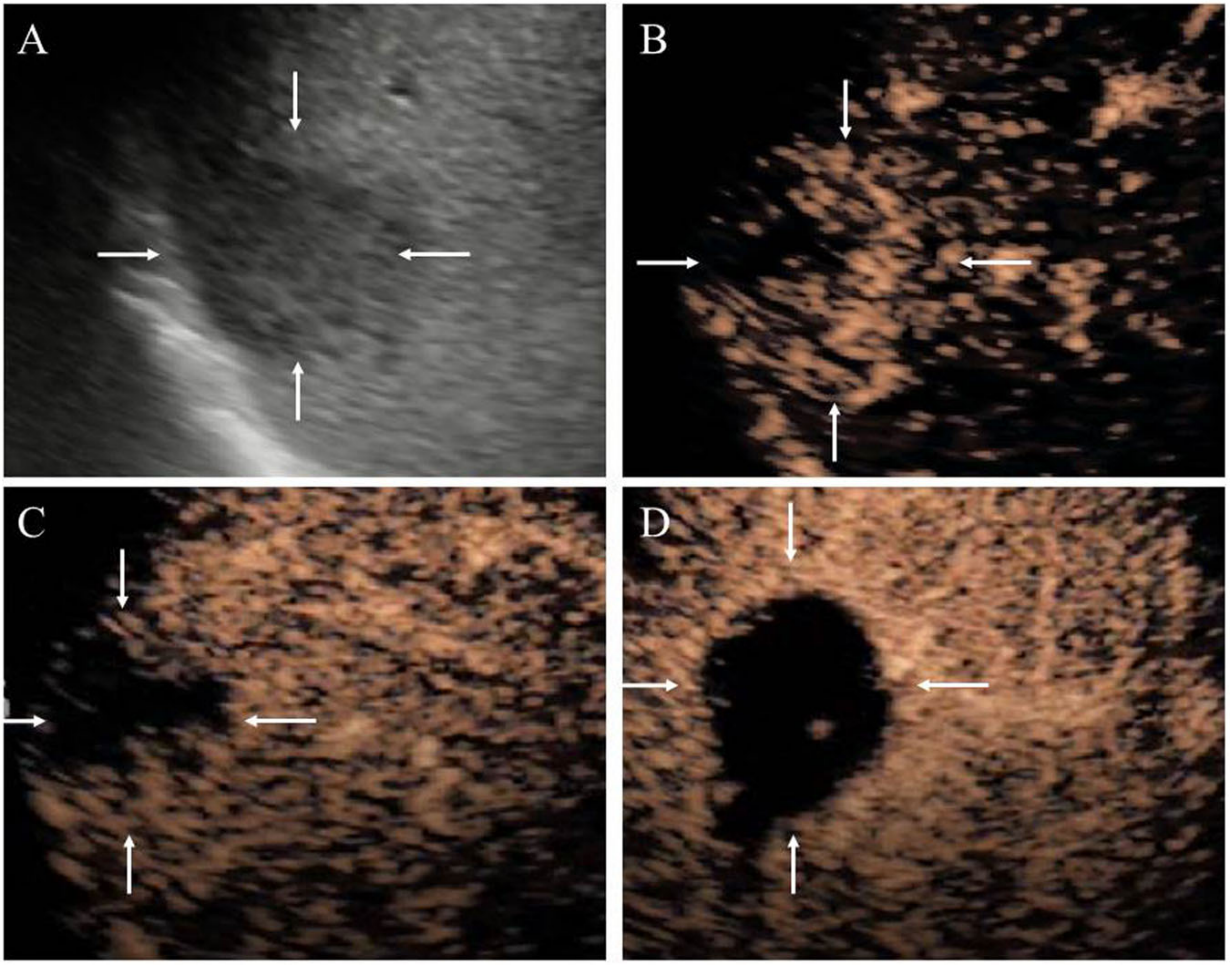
A 59-year-old woman with a 33 mm intrahepatic cholangiocarcinoma (ICC) lesion which is confirmed by pathology as SD-type. A B-mode ultrasound shows a hypo-echoic lesion (white arrow) with an ill-defined boundary located in the peripheral area. B Arterial phase rim hyperenhancement (rim-APHE, white arrow) is observed at 22 s after contrast agent injection. C Early wash-out is observed at 38 s (white arrow). D Marked wash-out is present at 65 s (white arrow)
